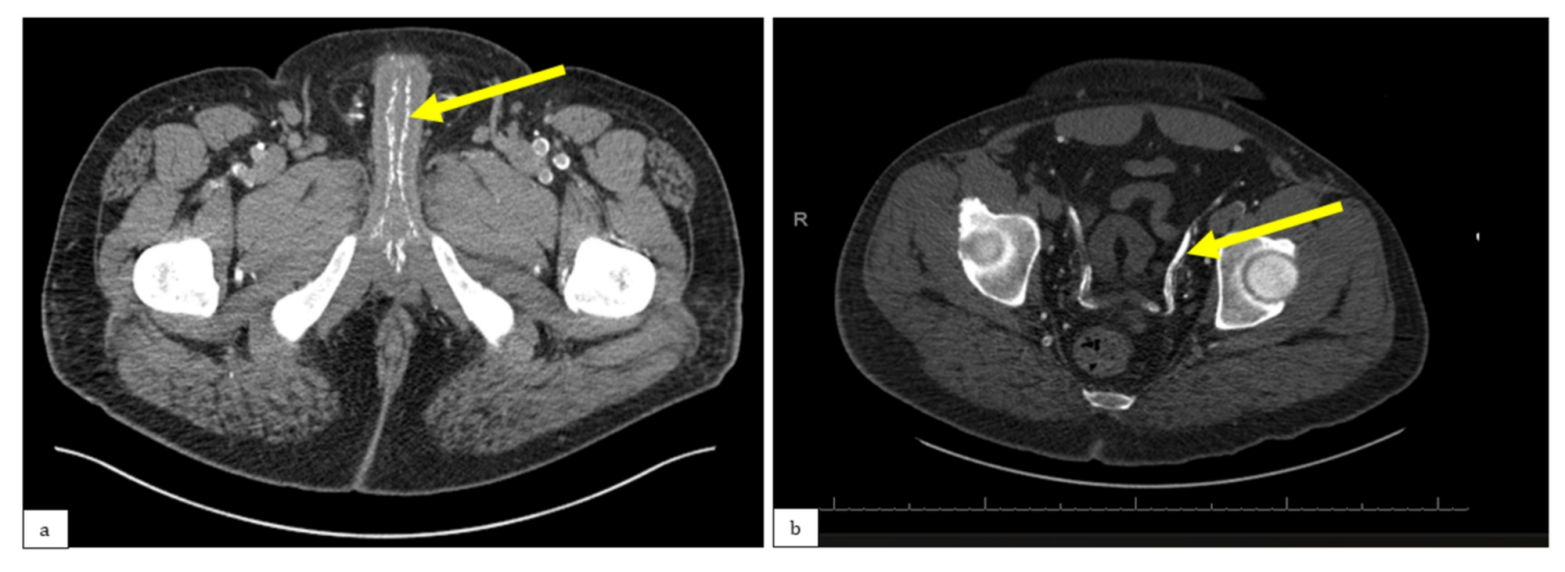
a) Non-contrast CT scan showing extensive calcification of the cavernosal arteries (yellow arrow); b) non-contrast CT scan showing calcification of the vas deferens (yellow arrow) CT: computed tomography
